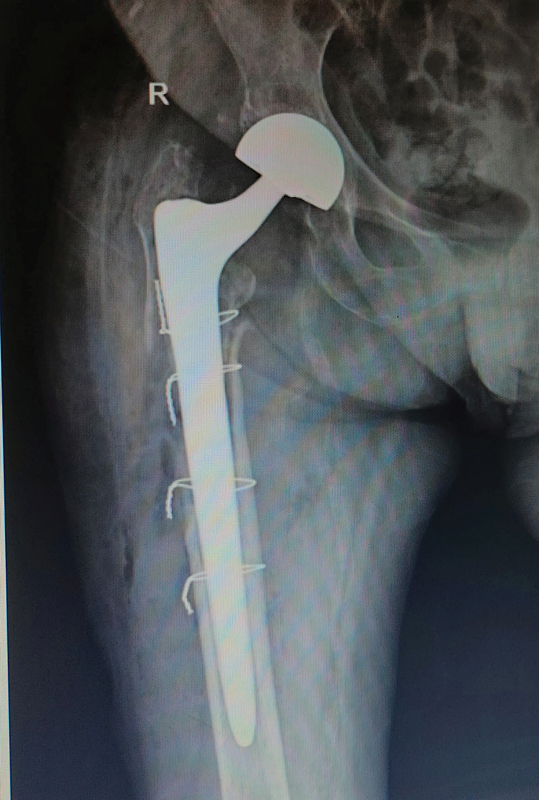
Immediate postoperative X-ray on AP view.
